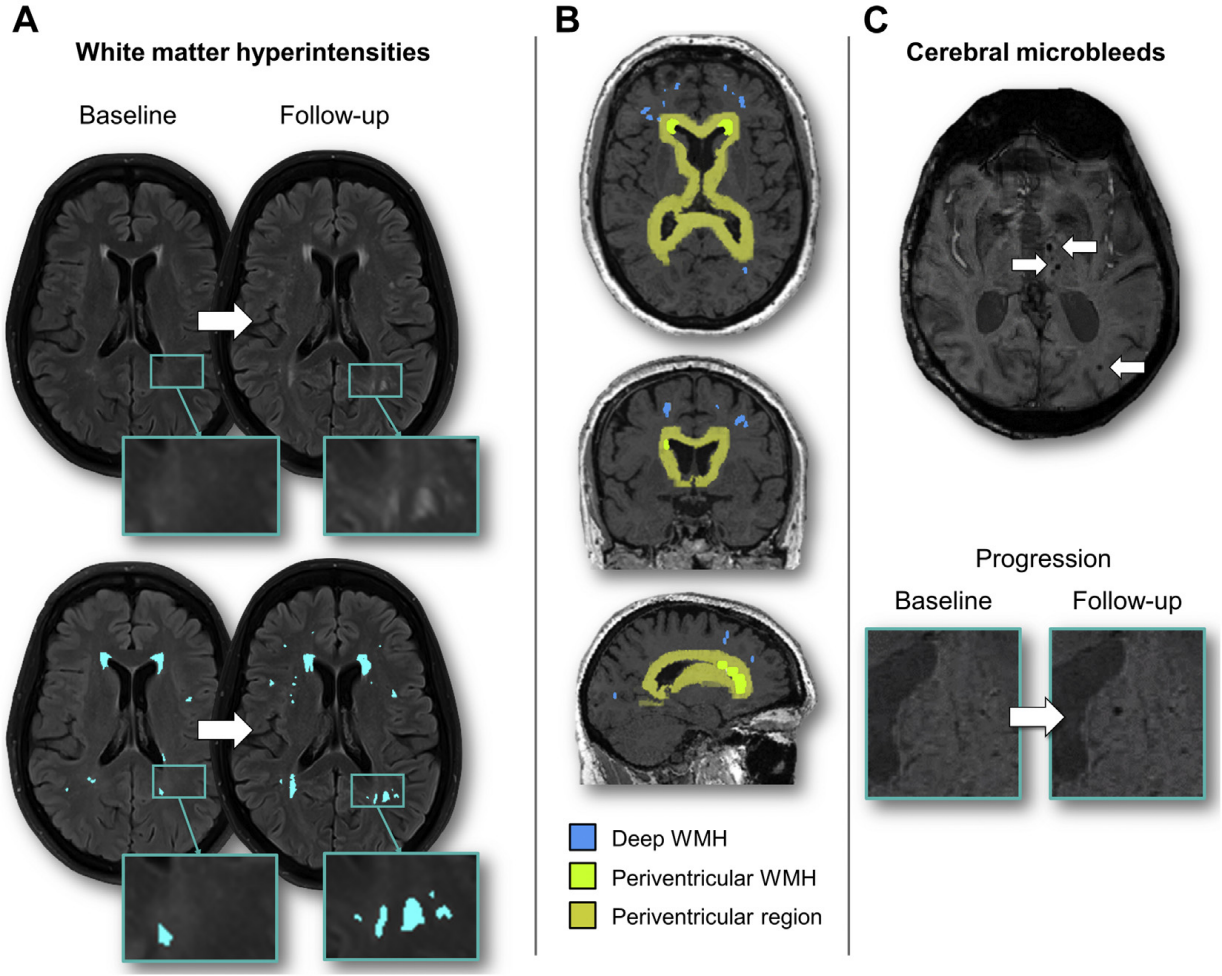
Radiological markers of cerebral small vessel disease (SVD). (A) White matter hyperintensities (WMH) were manually delineated on FLAIR MRI at baseline and two-year follow-up; (B) Classification of periventricular and deep WMH based on threshold distance from ventricles, derived from morphological ventricle dilation; (C) Cerebral microbleeds were identified on 3T SWI scans, and progression was defined as the occurrence of new microbleeds at follow-up, which were confirmed to be absent at baseline.
